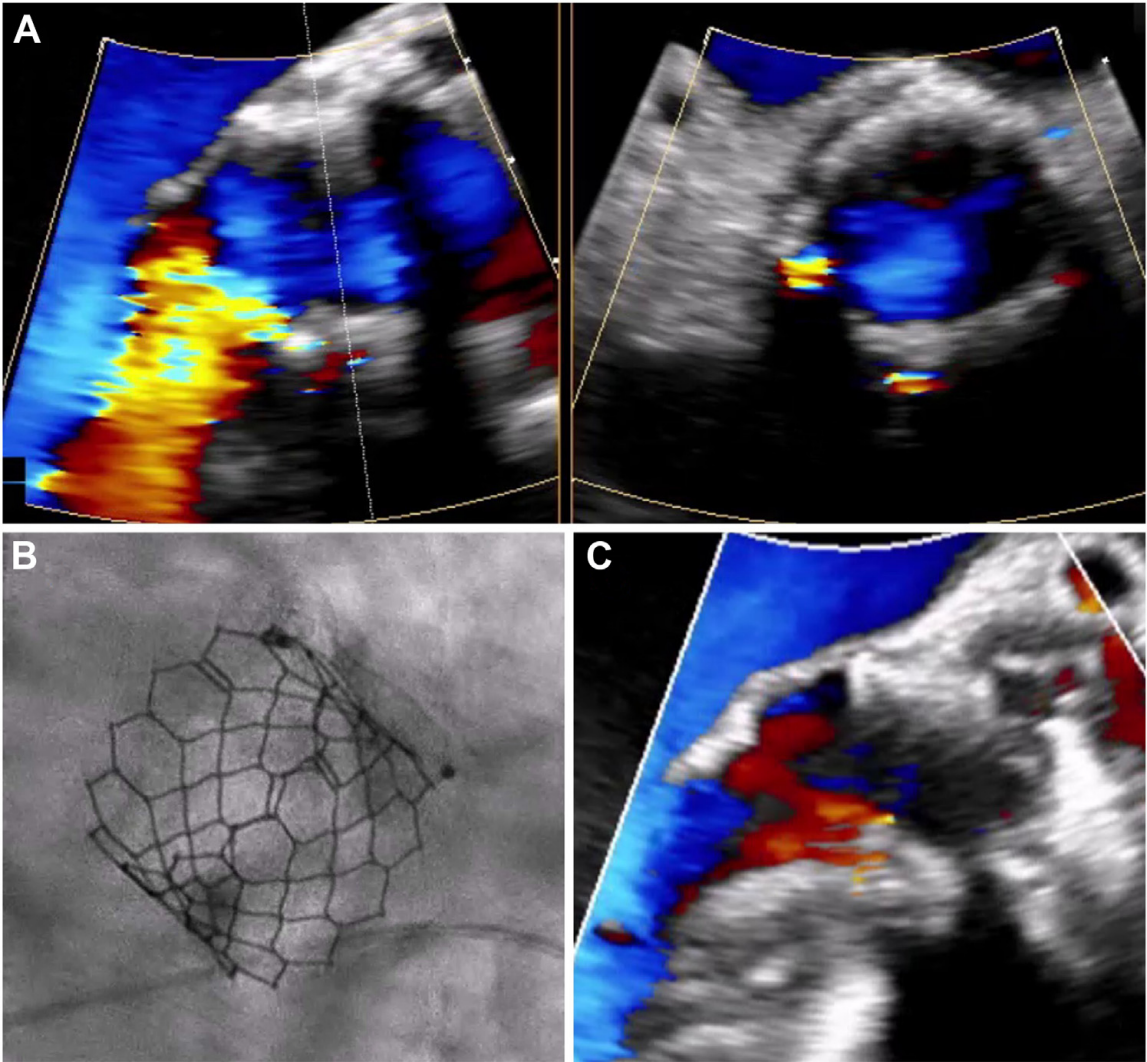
Case 2 Paravalvular Leak Evaluation and Final Result (A) Transesophageal evaluation of paravalvular leak evidencing a mild paravalvular leak. (B and C) Final angiographic and echocardiographic result after percutaneous plugging.
